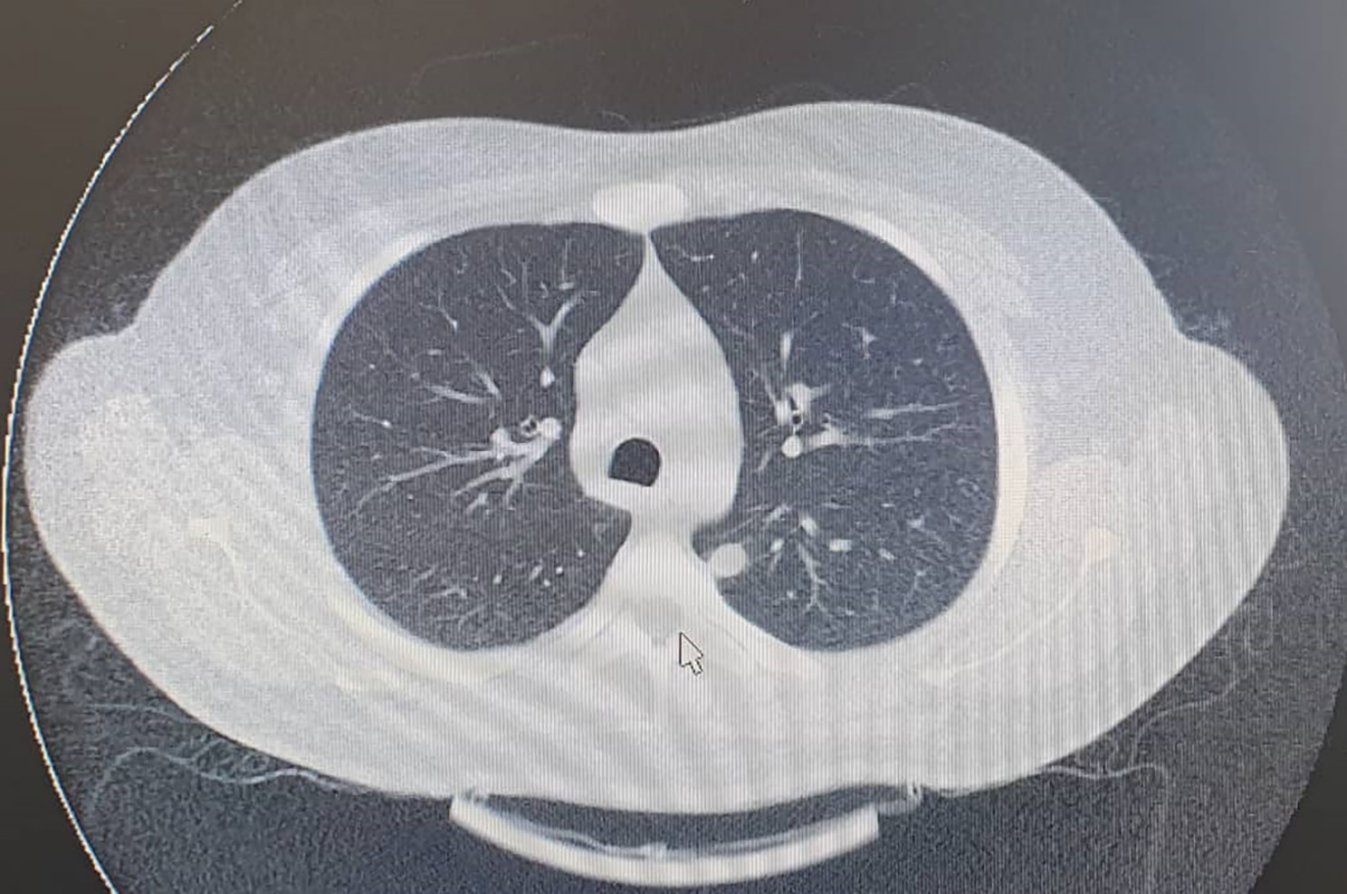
A feature of pulmonary nodules in CT scanning.
